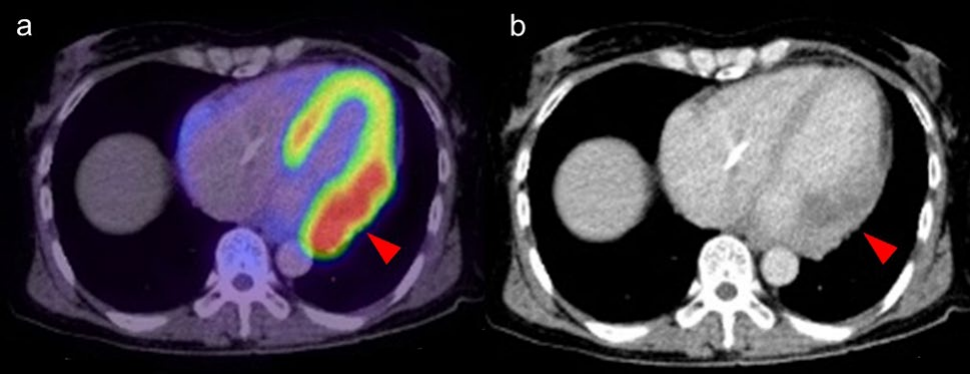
Primary cardiac lymphoma. Intense FDG uptake at left lateral wall was pathologically diagnosed diffuse large B cell lymphoma
